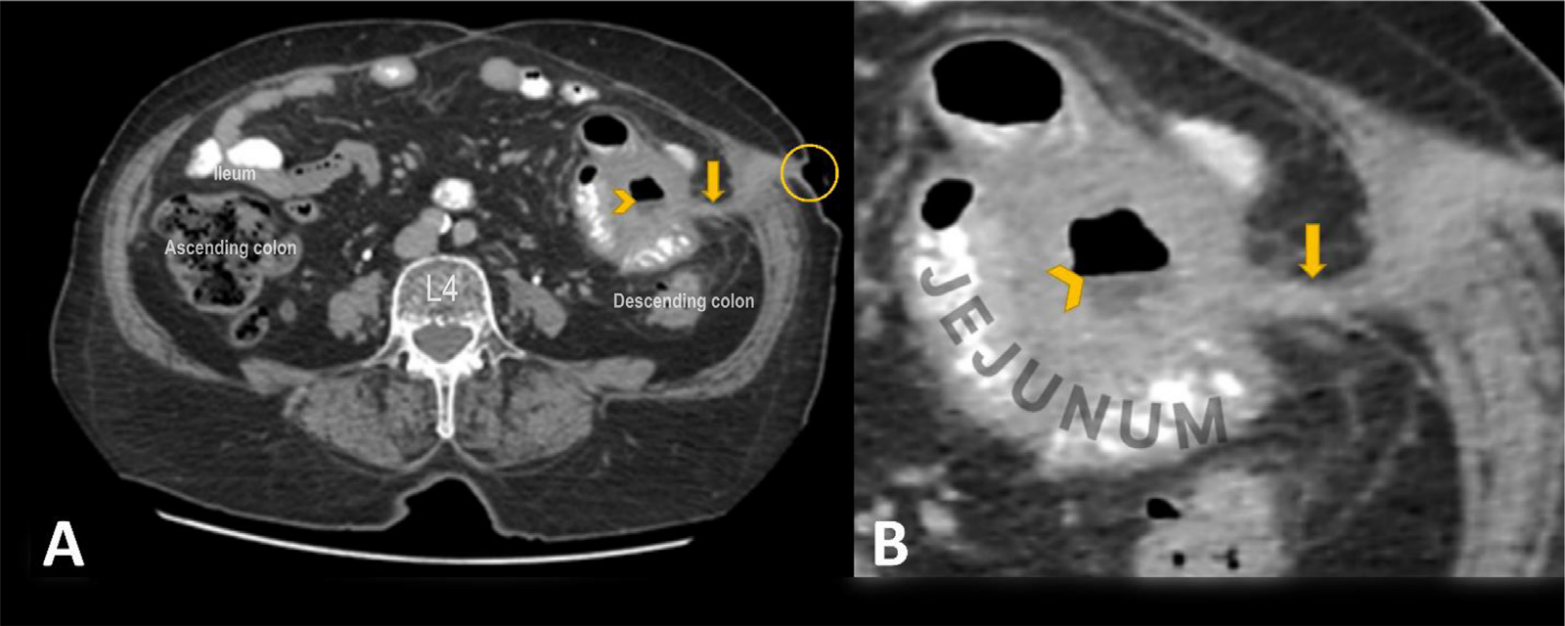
(A) Axial abdominopelvic CT scan with intravenous (IV) and oral (PO) contrast showing a 55-mm fistulous tract (arrow) connecting peritoneal collections and the anterior abdominal wall in the left lower quadrant (LLQ), adjacent to the jejunoileal loops (the skin orifice is marked by a circle). A peritoneal collection measuring 22 × 16 mm is also noted (arrowhead). (B) Magnified view of panel A highlighting the fistulous tract and associated findings.
